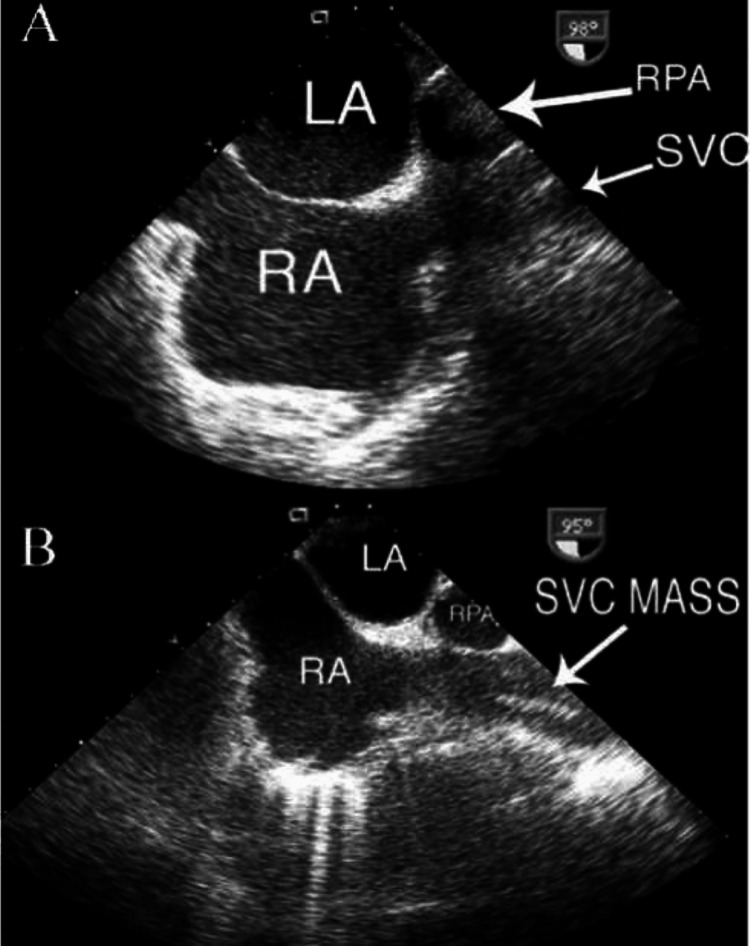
Echocardiography showed a large mass, most likely a thrombus, at the SVC-RA junction (arrow). SVC-RA: superior vena cava-right atrial
